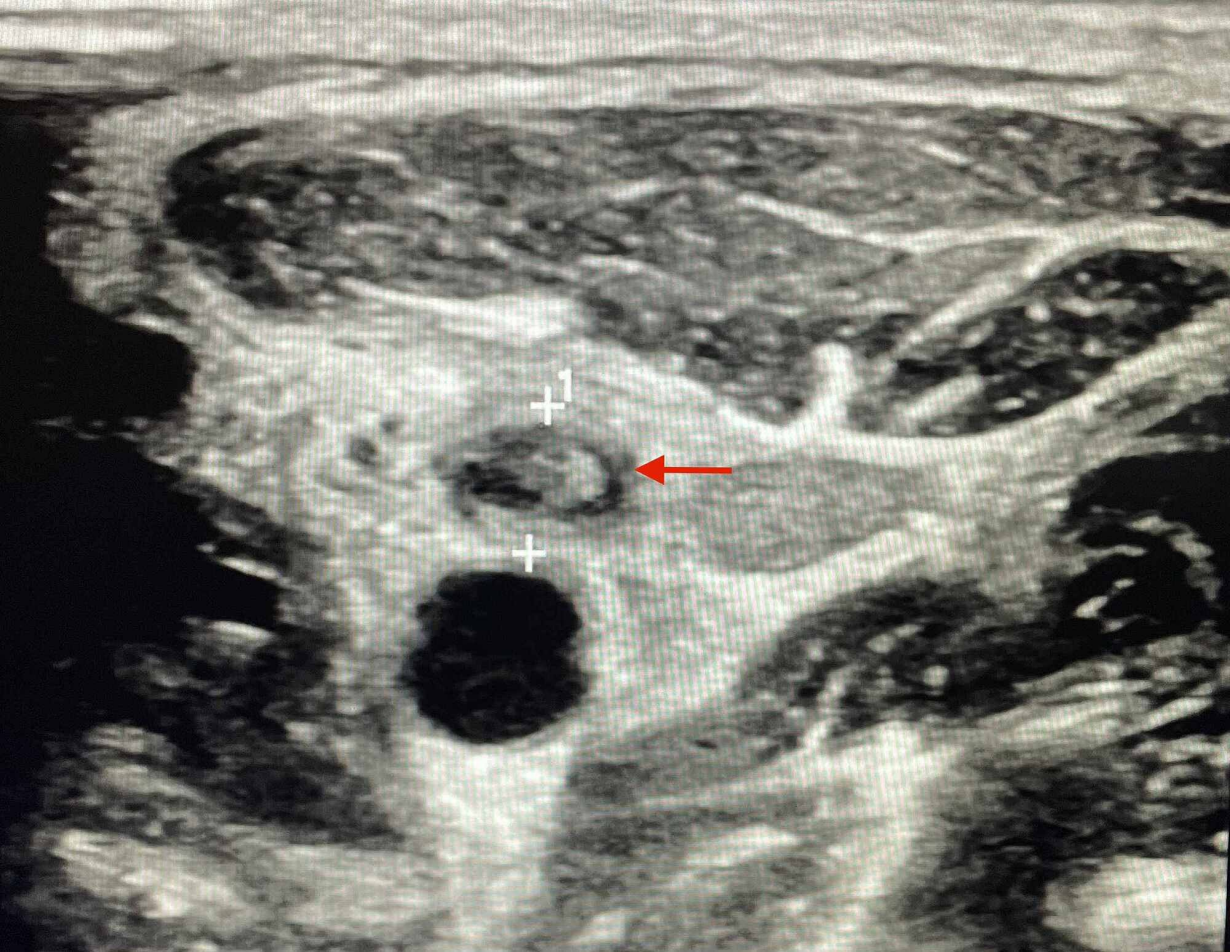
Neck ultrasound showing left internal jugular vein thrombosis (red arrow).
